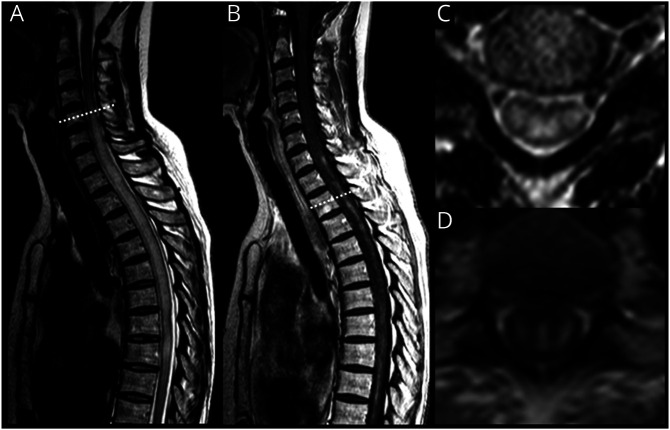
Spinal MRI in Subacute Rapidly Progressive HAM Demonstrating Enhancement A 54-year-old woman with subacute HAM presenting with a 1-month history of rapidly progressive paraplegia and bladder dysfunction. Sagittal T2w and contrast-enhanced T1w MR images (A and B) of the cervicothoracic cord shows longitudinal intramedullary hyperintensity and associated longitudinal enhancement. Axial T2w image at the upper thoracic cord (C) level shows high T2w signal and swelling involving gray and white matter but most severe in the central cord. Axial contrast-enhanced T1W (D) shows central and peripheral enhancement in the region of the lateral corticospinal tracts, which mimics the “trident sign” seen in neurosarcoidosis.
